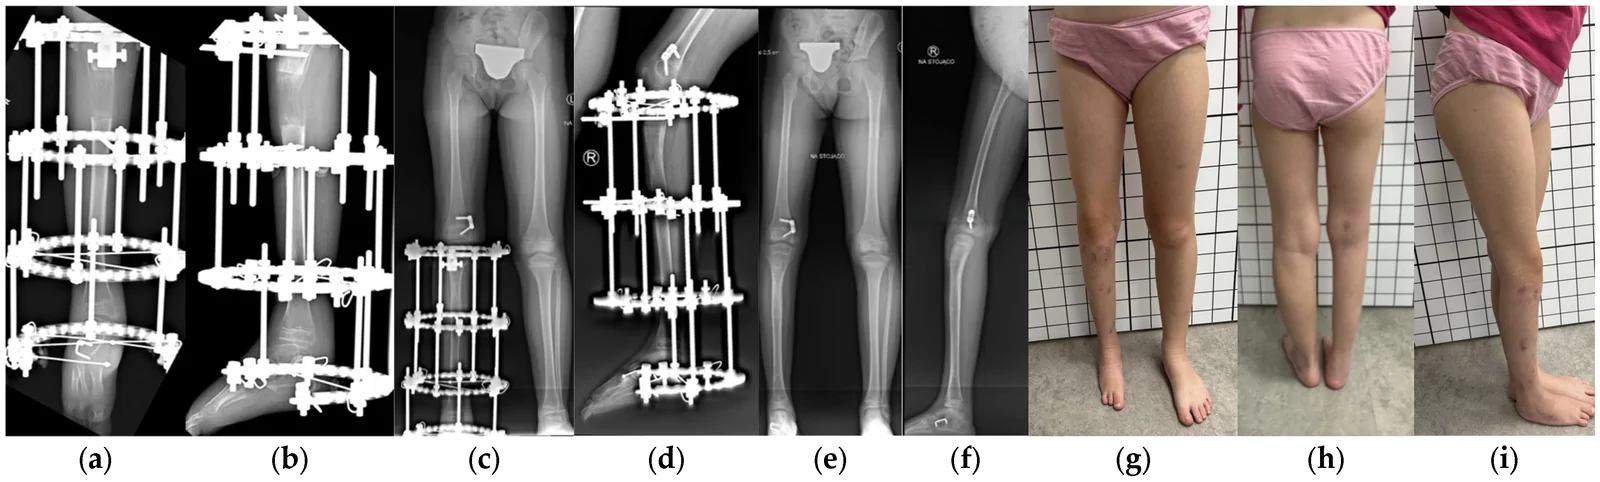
Limb lengthening in the same patient: (a,b) first lengthening using the Ilizarov method with foot fixation; (c,d) consolidation phase after 5 cm lengthening; (e,f) radiographic result; (g–i) clinical result. R—right lower limb, NA STOJĄCO—standing position.
